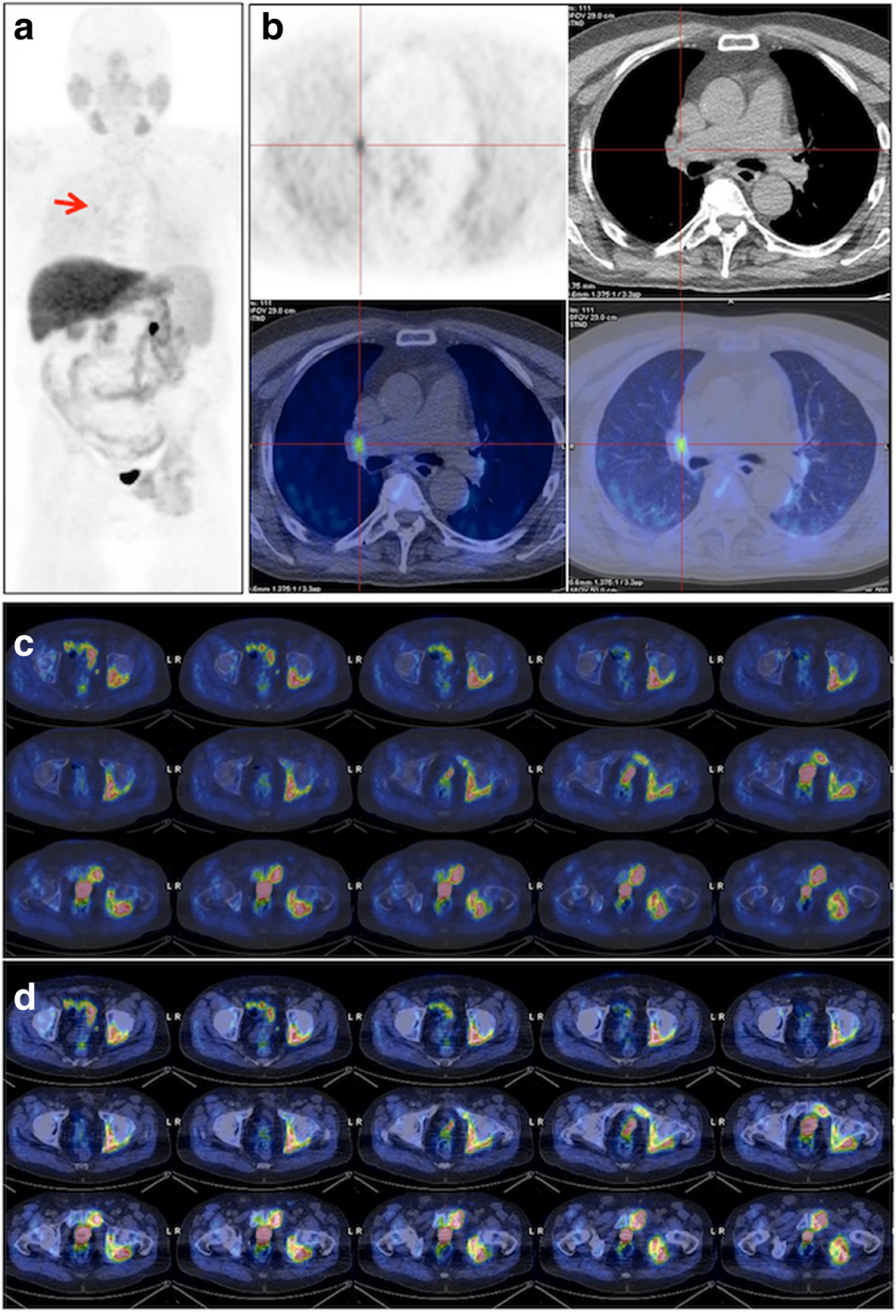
Case#1, 72-yr old man with prostate cancer treated with radical prostatectomy with radiological evidence of bone metastasis. [^18^F]fluorocholine PET/CT shows tracer uptake in the peri-hilar right lung region (a, MIP arrow) and in the left side of the pelvis. Transaxial images (b) confirm a metabolically active area in a peri-hilar right lung nodule subsequently diagnosed as non small cell lung cancer. Superimposed transaxial PET/CT images (c, bone window; d, soft tissue window) show [^18^F]fluorocholinee uptake in a vast metastatic lesion involving the left pubis, the left ischium and extending into the adjacent soft tissues. Bone metastases biopsy resulted inconclusive (metastases from adenocarcinoma of unknown origin)
